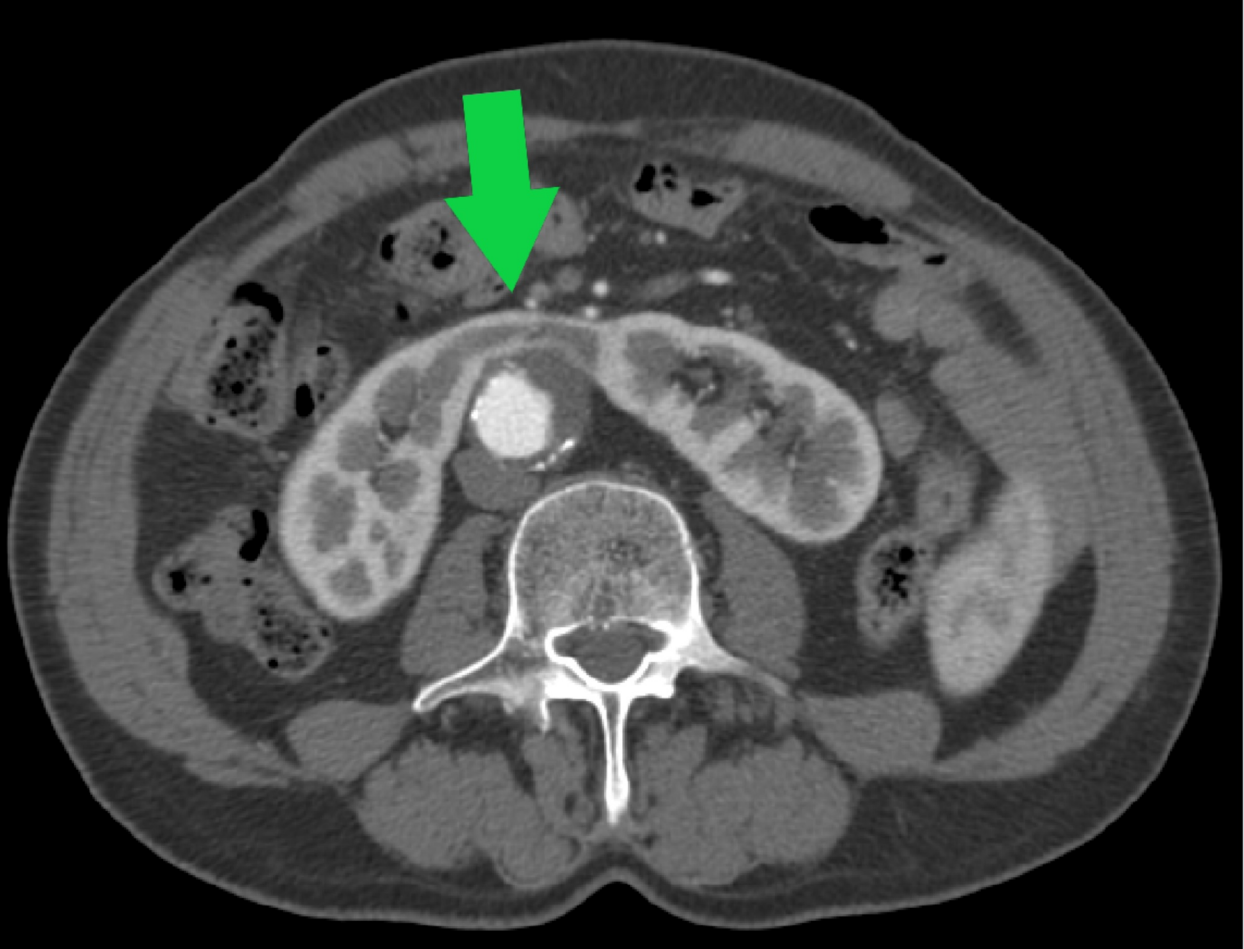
Preoperative abdominal CT angiogram demonstrating appropriate isthmus perfusion (green arrow)
